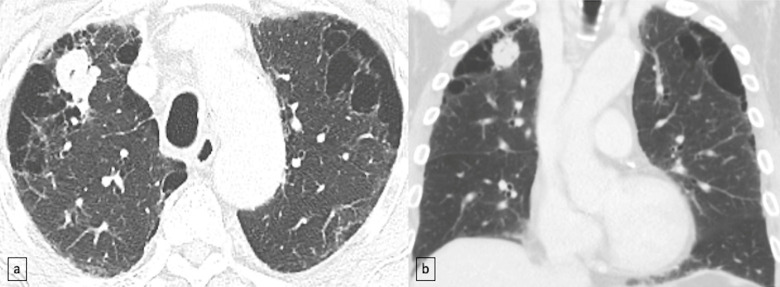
Squamous cell carcinoma and paraseptal emphysema. Axial (a) and coronal (b) CT images demonstrate right upper lobe squamous cell carcinoma and severe upper lobe paraseptal emphysema.
